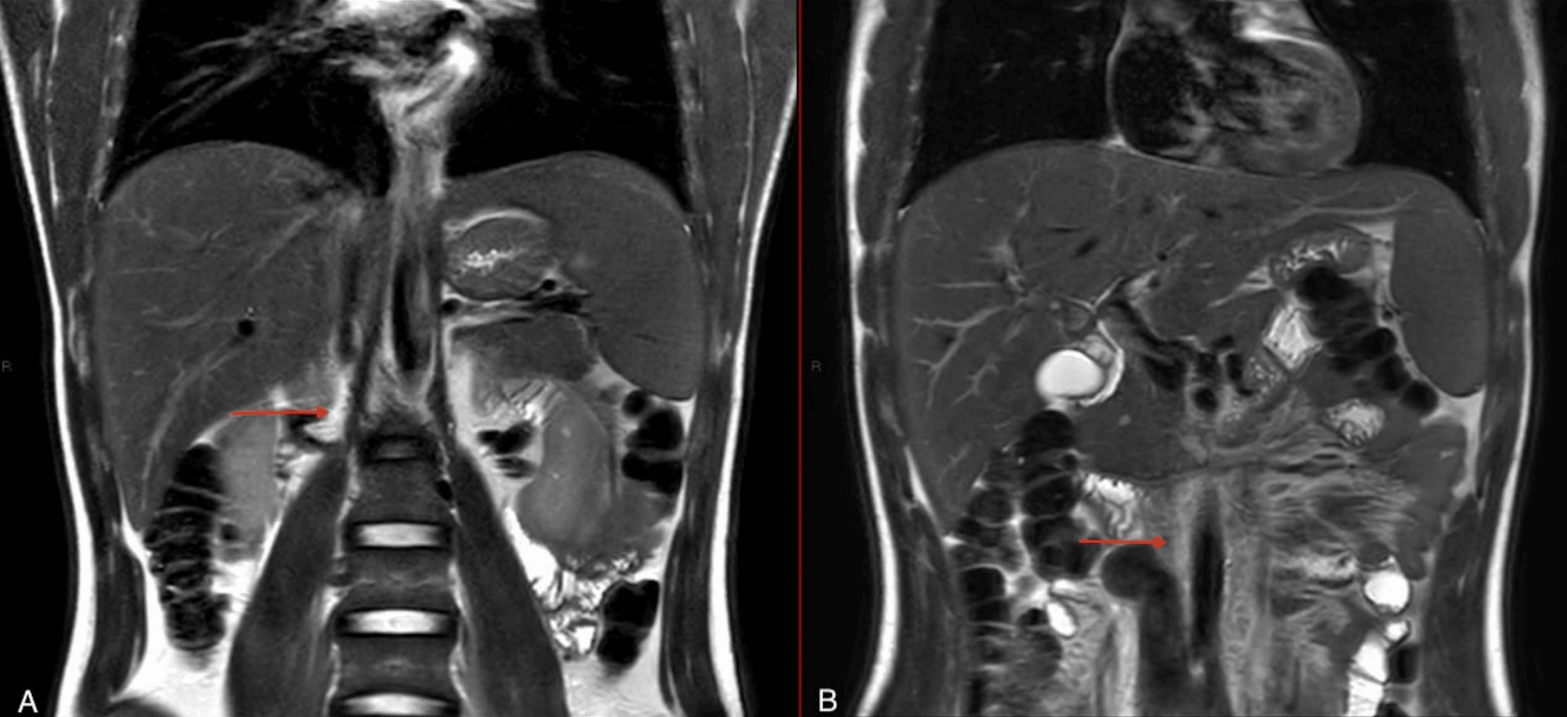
T2-weighted MRI abdomen without contrast - coronal view (A) Image showing atretic segment. (B) Image showing non-opacified dilated IVC. IVC: inferior vena cava
